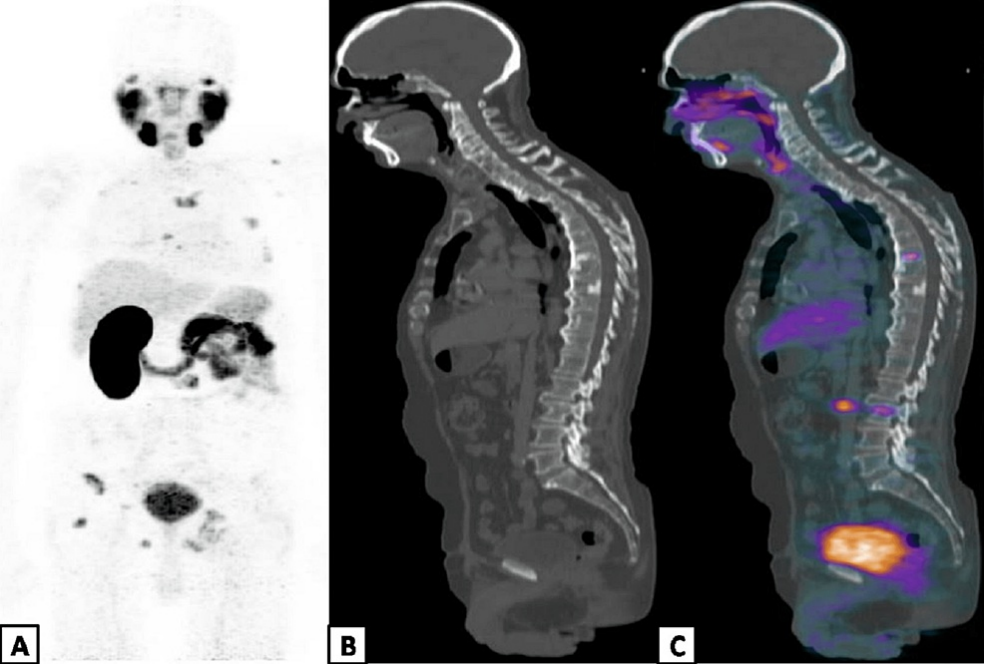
PSMA PET-CT scan Maximum intensity projection (A), sagittal CT (B) and fused sagittal PET-CT (C) demonstrated multiple osseous metastases.
